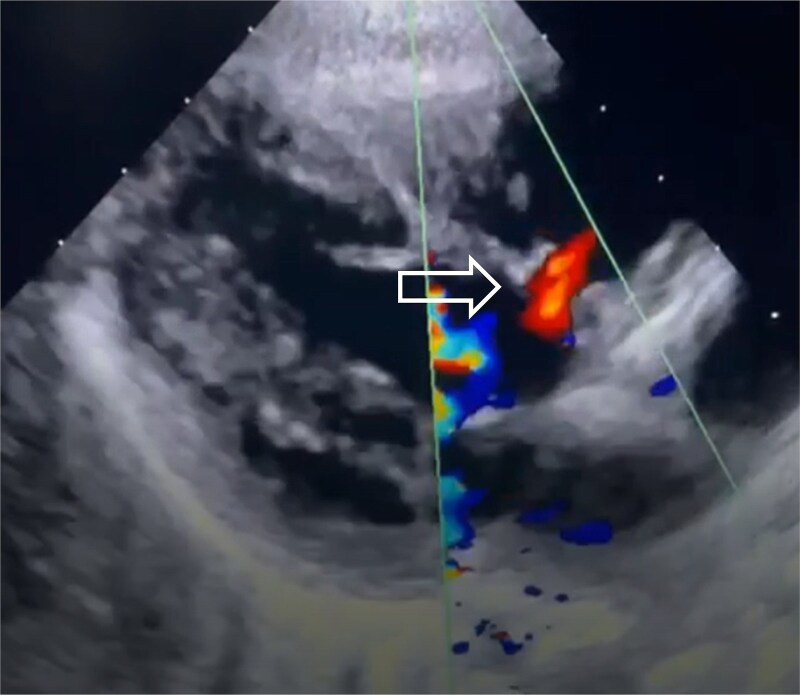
Baseline echocardiographic image demonstrating a moderate outlet muscular ventricular septal defect with a diameter of 4.5 mm.
